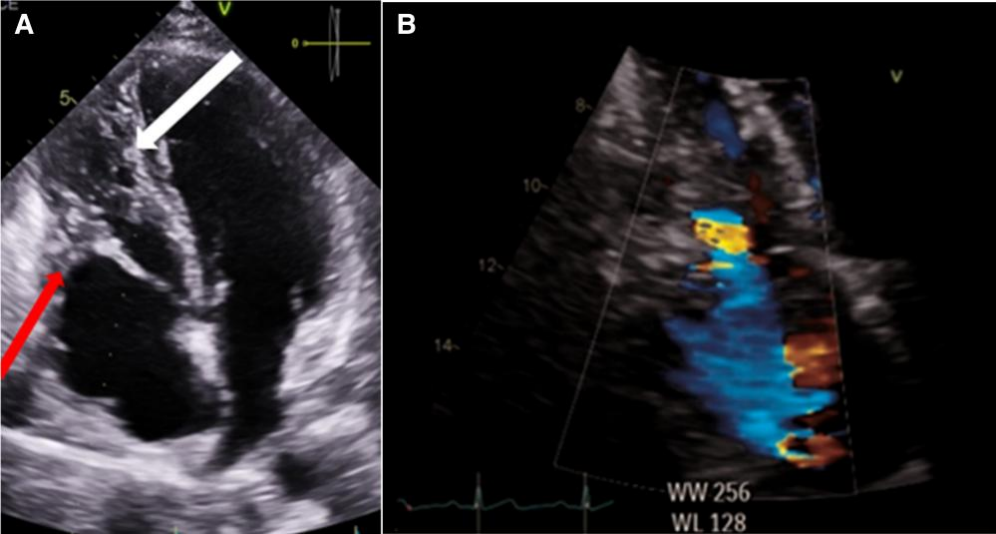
Apical four-chamber view in transthoracic echocardiography indicating (*A*) hypertrabeculation of the right ventricle (arrow), a high insertion of the posterior leaflet of the tricuspid valve ( arrow), a dilated right atrium—(*B*) moderate tricuspid regurgitation.
